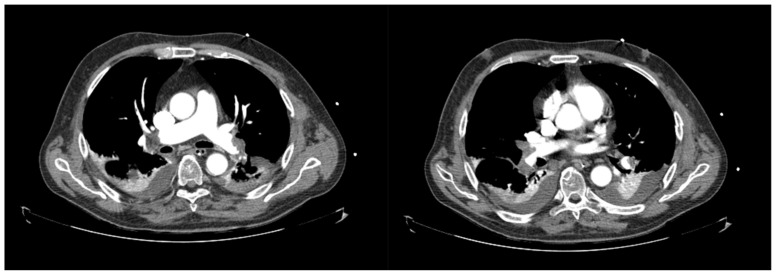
Post-procedural thoracic Angio-CT showing persisting large bilateral pulmonary embolism with significant thrombotic burden.
